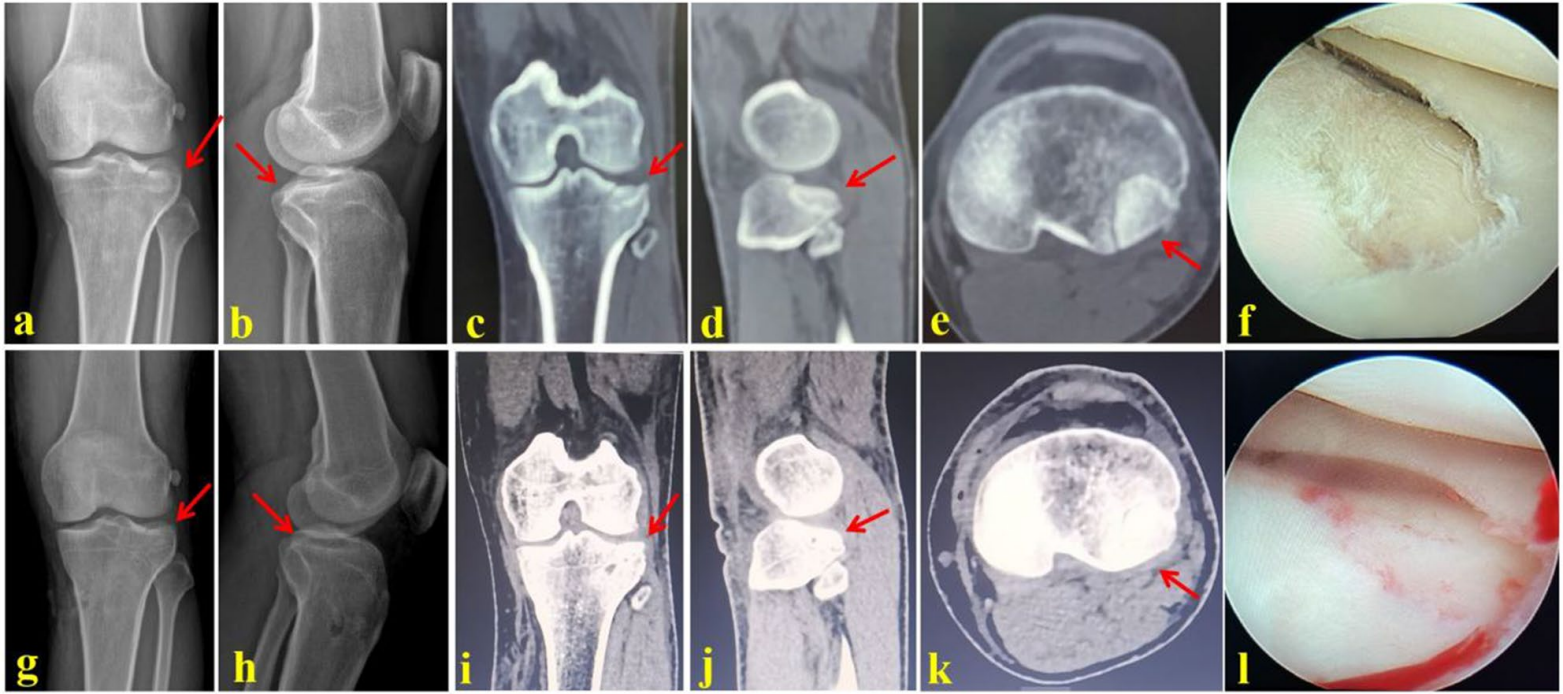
A 37-year-old male suffered from the PL-depression tibial plateau fracture and was successfully treated by the RA technique. (a,b) AP and lateral X-ray images of the injured tibial plateau; (c–e) Coronal, horizontal and sagittal CT scan images before operation; (f) Arthroscopic image of the PL-depression fragment before operation; (g,h) AP and lateral X-ray images of the tibial plateau after operation; (i–k) Coronal, horizontal and sagittal CT scan images after operation; (l) Arthroscopic image of the PL-depression fragment after operation. Red arrows indicate the PL-depression fragment of the tibial plateau.
